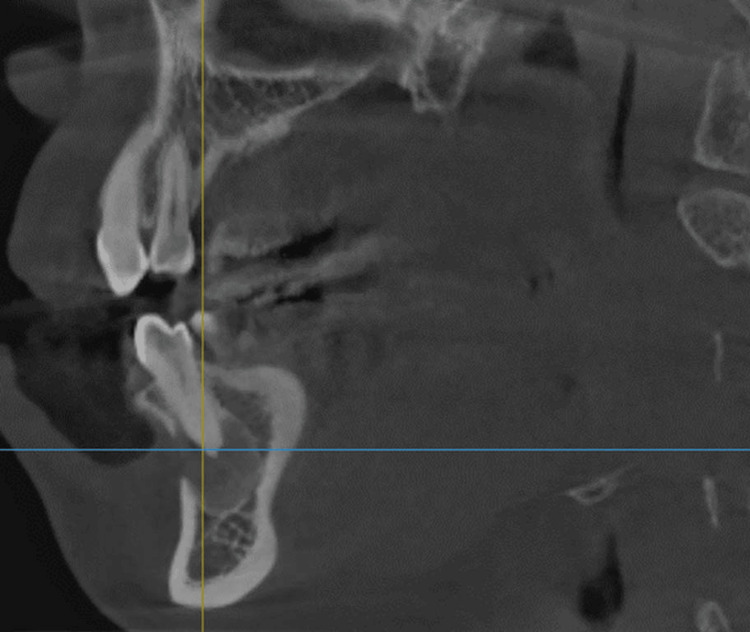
Dental cone beam computed tomography (CBCT) of the lesion A single, well-demarcated lesion with buccal cortical destruction was observed in the sagittal view.
